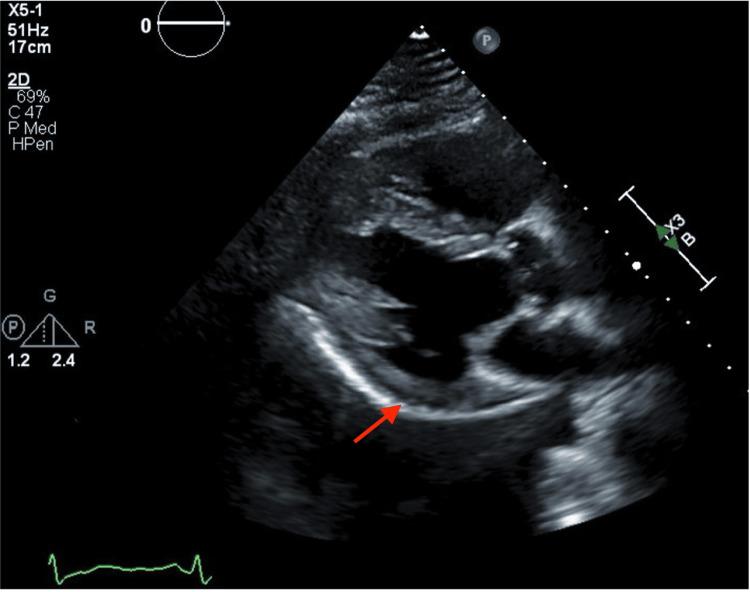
Parasternal long-axis echocardiographic view demonstrating inferolateral left ventricular hypokinesis, correlating with ischemia in the affected myocardial territory
